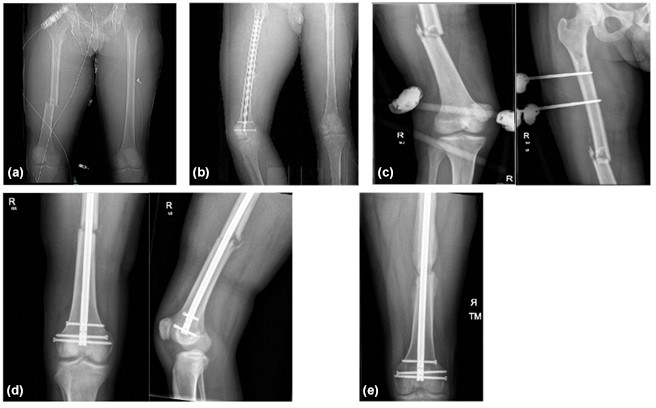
Figure 1(a) Initial CT scan showing the femur fracture. (b) CT scan following initial fixation with intramedullary nailing. (c) Plain radiograph following temporary stabilisation with a knee-spanning external fixator following removal of nail and bony debridement. (d) Plain radiograph following revision fixation with removal of an external fixator and intramedullary nailing. (e) Plain radiographs at 8-month clinic follow-up showing a bridging callus across the fracture site.
